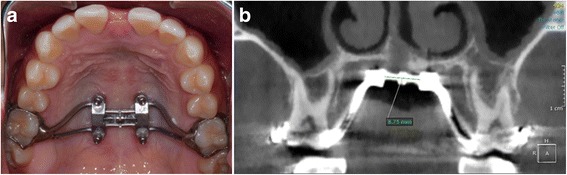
Maxillary Skeletal Expander (MSE). a Appliance positioned in the posterior part of the palate. b Measurement of the distance between the two halves of the expansion jackscrew on the post-expansion CBCT; the opening of the midpalatal suture can also be seen in the figure
